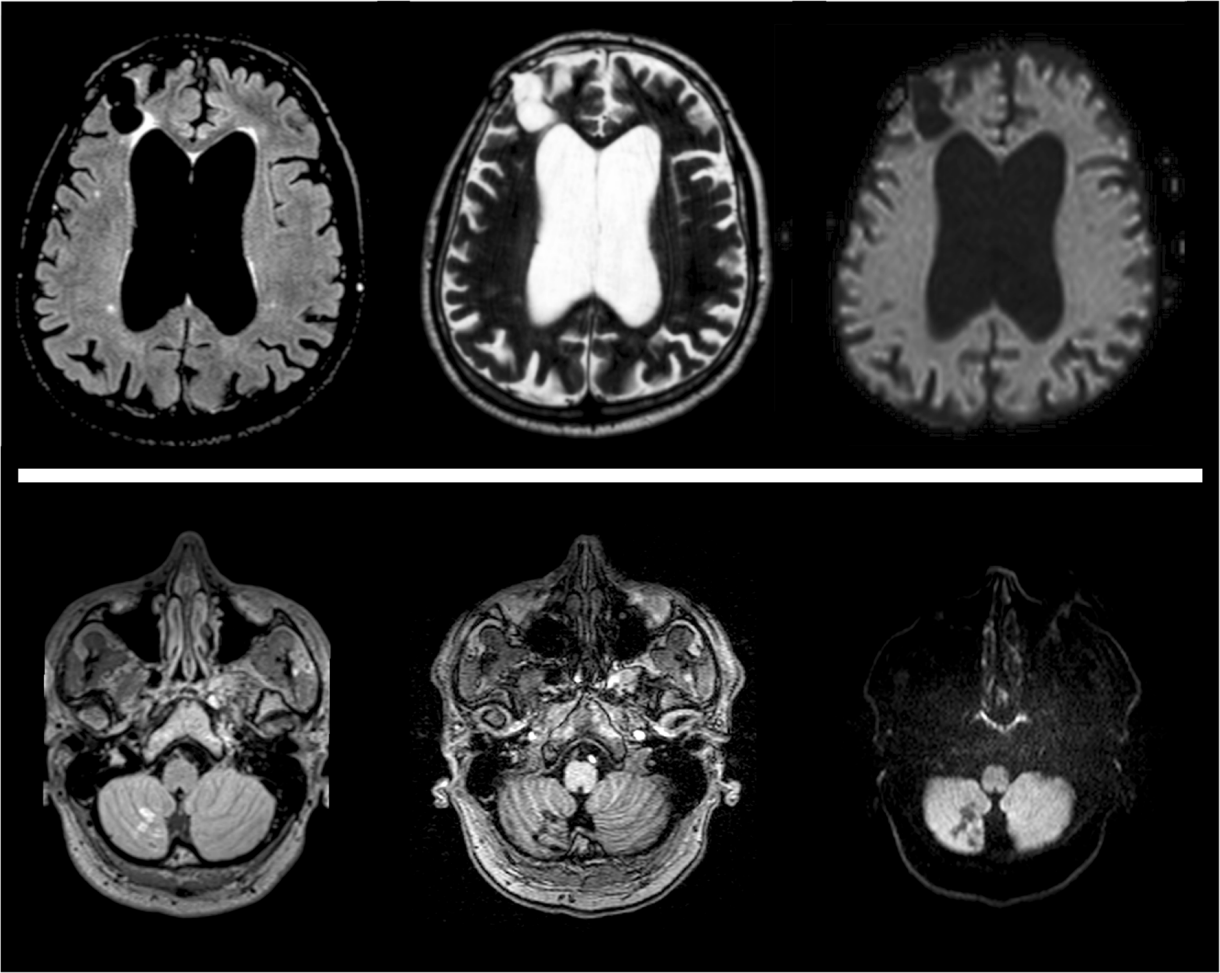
Brain MRI findings in Fabry disease patients suffering from major cerebrovascular events. In the *upper row*, images obtained from a 61-year-old woman showing the presence of a large chronic ischaemic lesion at the level of the right frontal lobe, coupled to a significant enlargement of lateral ventricles, while in the *lower row* small ischaemic lesions at the level of the inferior portion of the right cerebellar lobe are present in 52-year-old man
